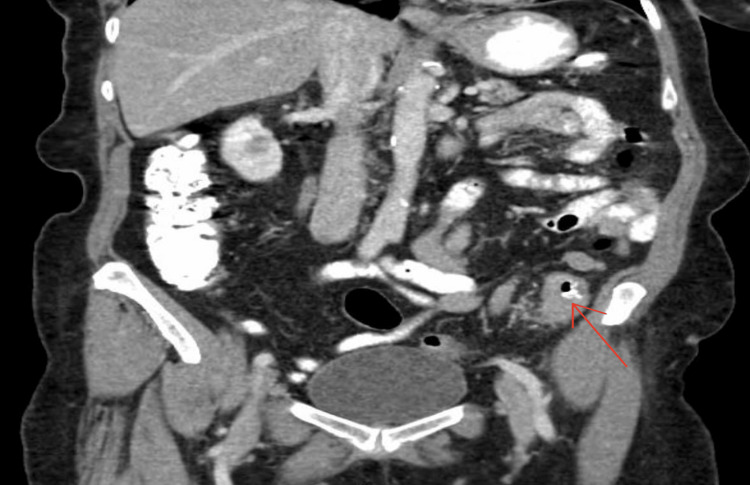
Coronal CT scan of the abdomen shows a thickened segment of the large bowel (red arrow)
